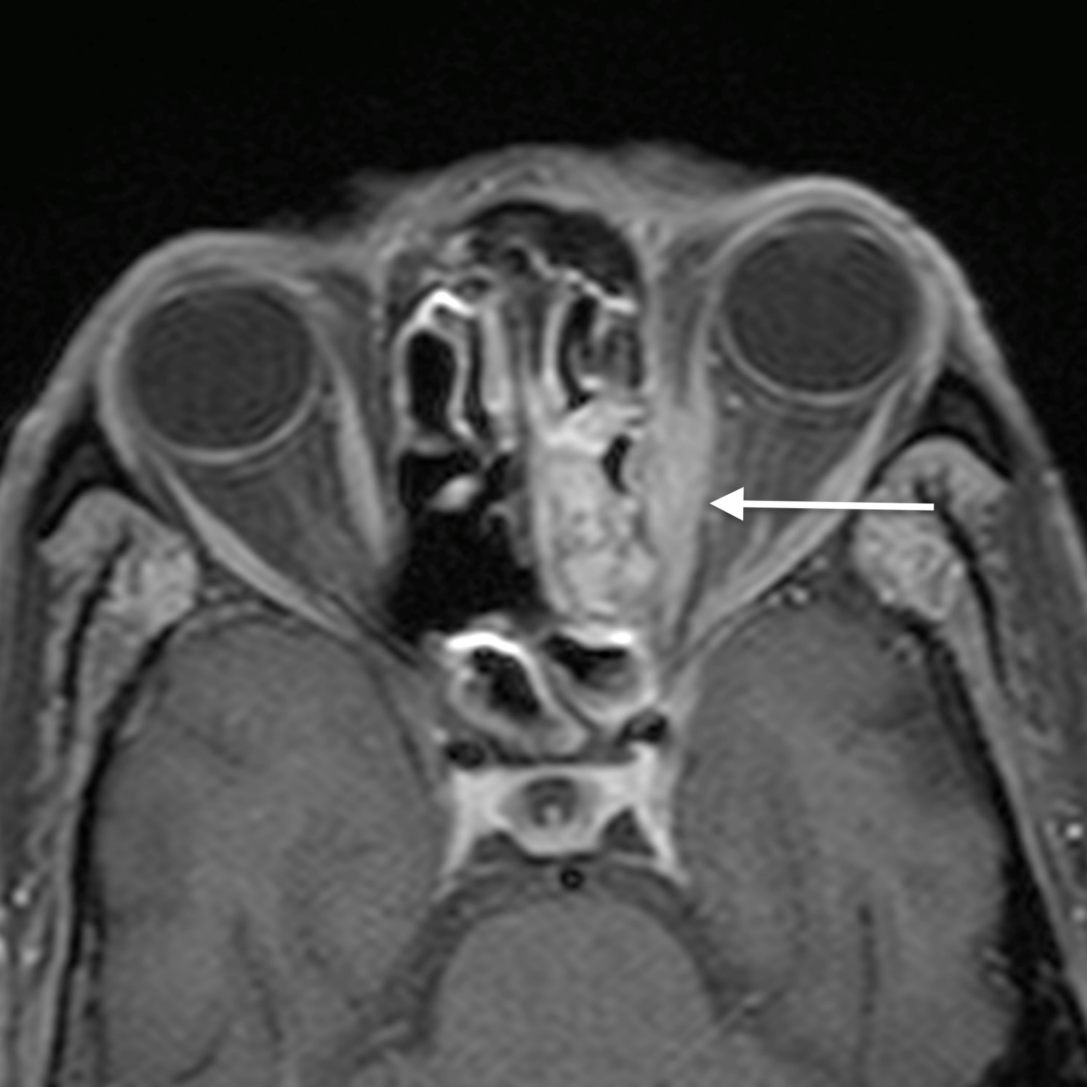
Orbital cellulitis: axial post-contrast T1WI shows enhancement of the abnormal soft tissue (arrow) T1WI - T1-weighted image
